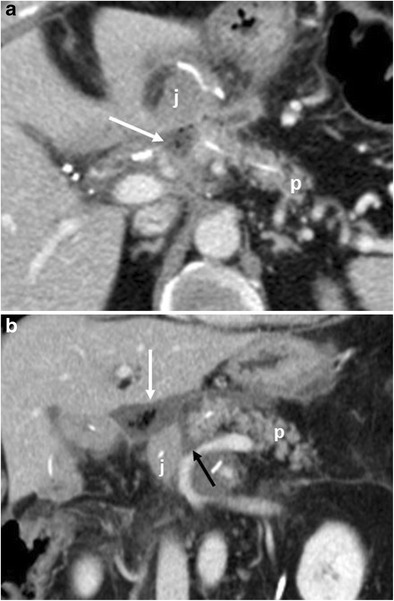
Pancreatic fistula. a, b Multiplanar CT images. The pancreatic stump (p) and jejunal loop (j) are visualised. A complete disruption of the pancreatic anastomosis is evident (black arrow in b). At the level of the anastomosis a fluid collection with multiple air bubbles inside is visible (white arrows), a finding that strongly suggests the presence of a pancreatic fistula
